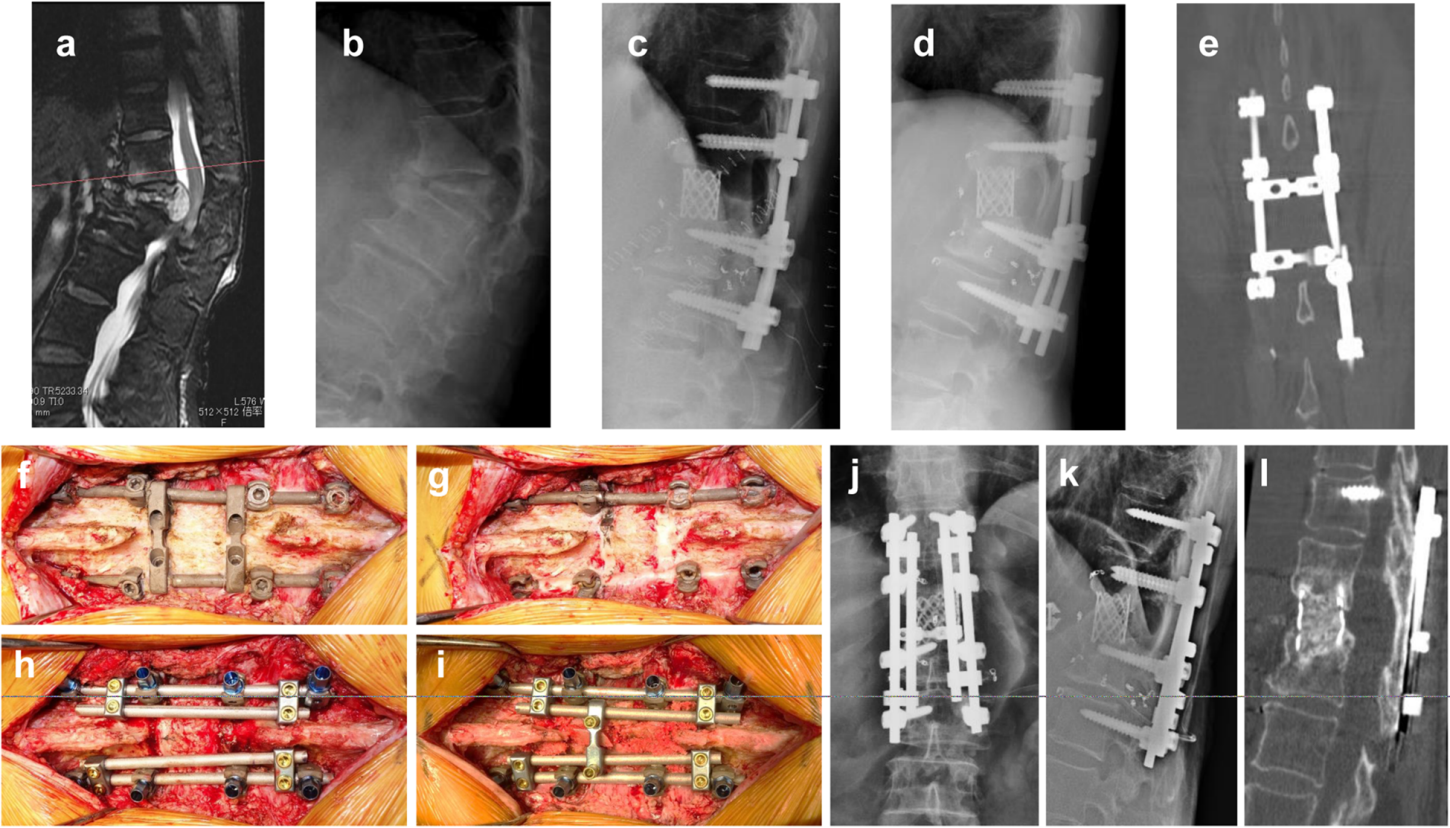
Case 18. A 66-year-old woman with breast cancer metastasis at L1. a Preoperative sagittal T2 magnetic resonance image revealing tumor involvement at L1. b Lateral radiograph revealing pathological fracture at L1. c Lateral radiograph after total en bloc spondylectomy. d Radiographs at 18 months after surgery revealing increased local kyphosis angle between T12 and L2. e Coronal computed tomography (CT) scan revealing breakage of the left rod. f Posterior instrumentation is exposed using the previous midline incision. g The left rod is broken at the area proximal to the L2 pedicle screw. h Loosening pedicle screw at the left T11 level is replaced, and four cobalt chromium rods are inserted. i Autologous bone graft is placed on adjacent T12 and L2 lamina and scar tissue of the resected L1 lamina area. j, k Radiograph after revision surgery. l Sagittal CT scan at 42 months after revision surgery revealing bone fusion within the cage and at the posterior bone graft
